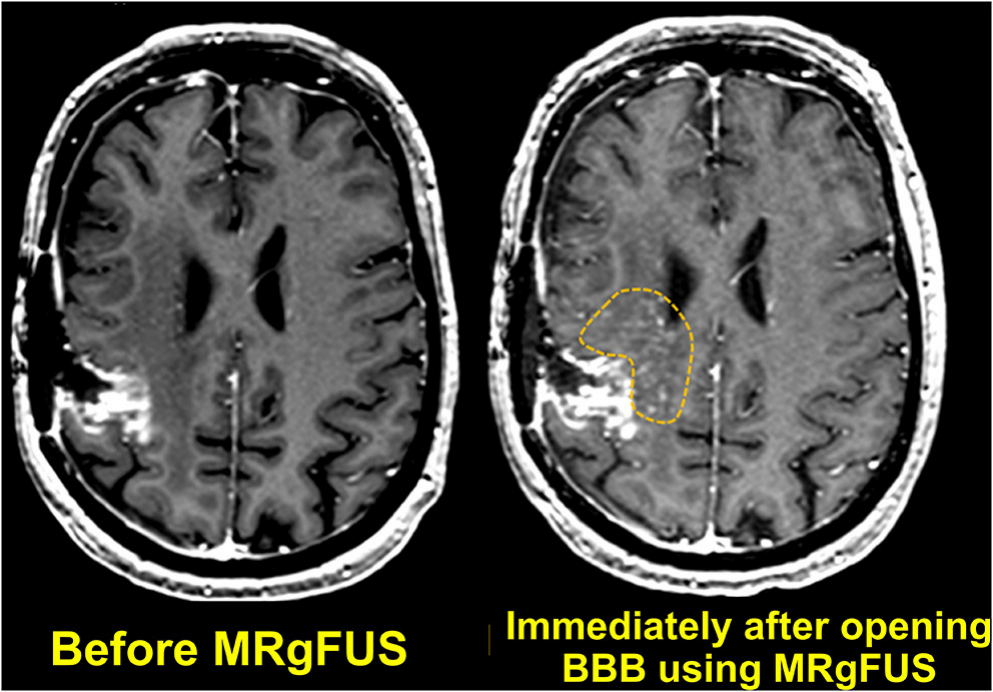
Axial T1-weighted MR images obtained before and immediately after MRgFUS was used to open the BBB. Localized extravasation (inside the dotted yellow circle) can be seen in the predefined targeted brain tissue.
